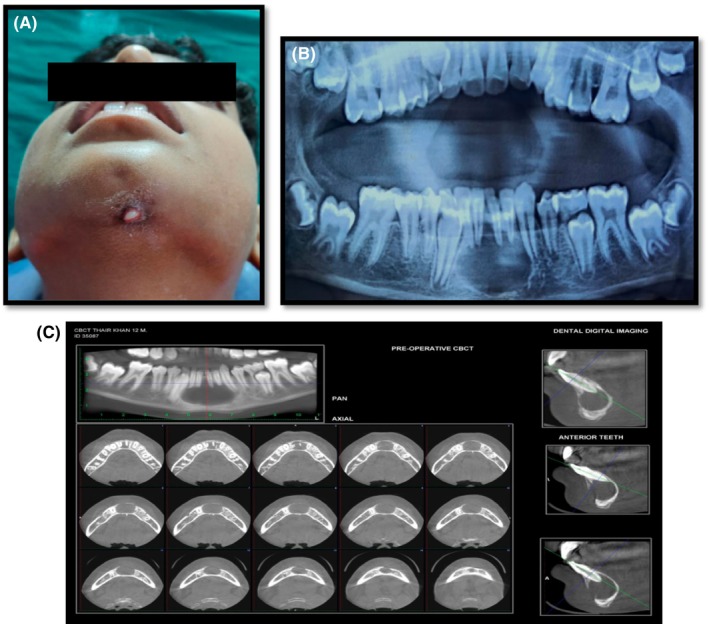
(A) Preoperative extraoral picture. (B) Preoperative radiograph.(C) Preoperative CBCT.
